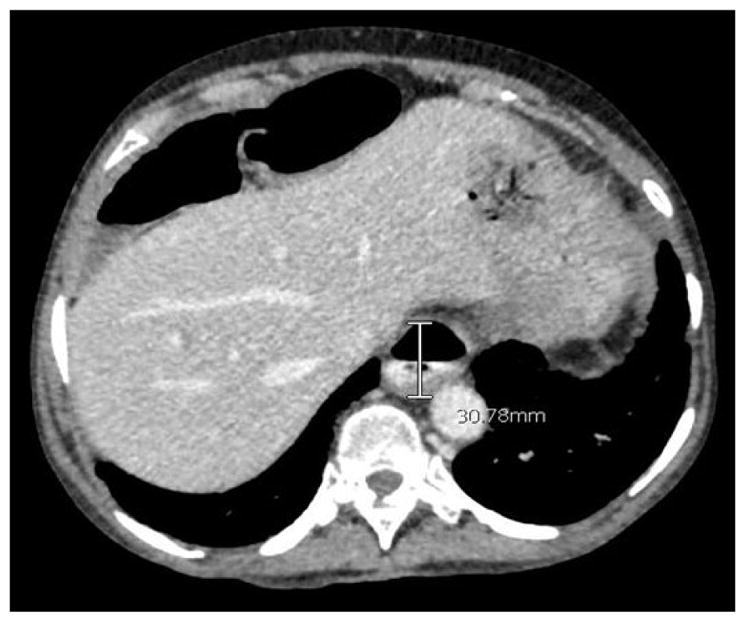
CT Abdomen with oral contrast in a patient with SSc demonstrates profound dilation of the distal lower third of the esophagus with 30 mm dilation and layer of intraluminal contrast.
